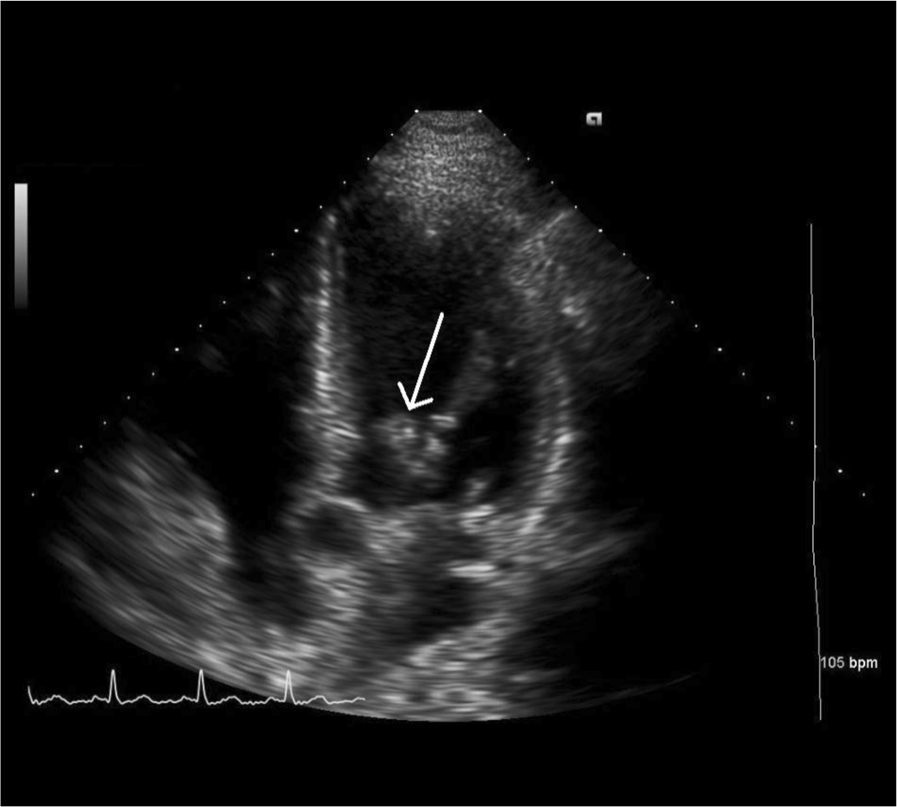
Transthoracic echocardiogram showing a mitral valve leaflet vegetation (arrow).
